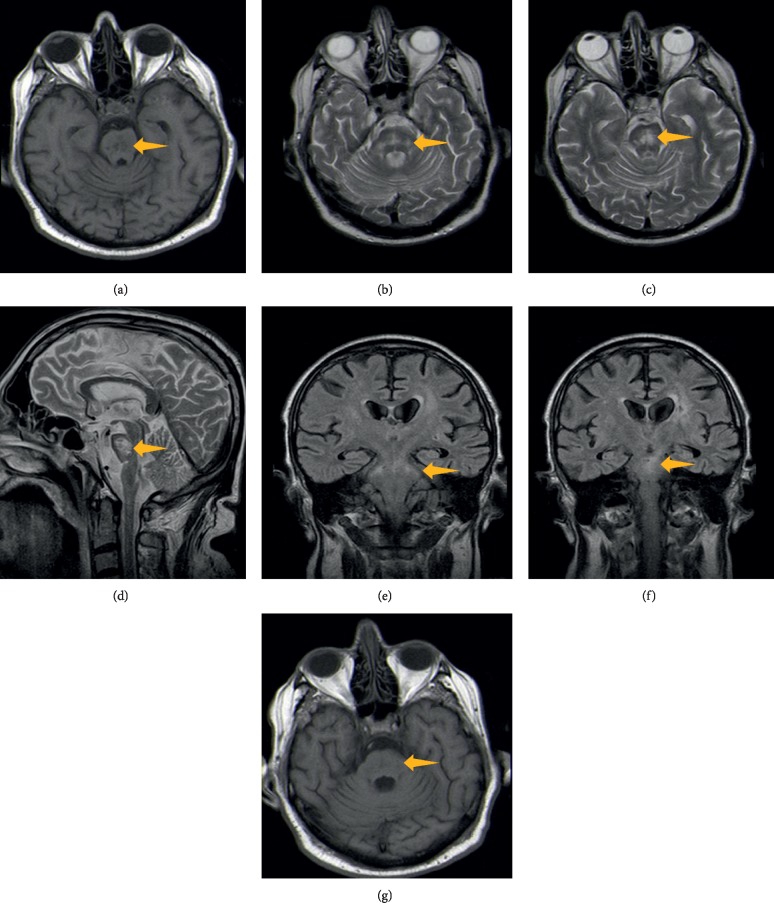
MRI images show central pontine T1 moderately hypointense areas (a), T2 and FLAIR areas of hyperintensity at the level of the pons (b, c, d, f), including an area trident-shaped of hyperintensity at the level of the pons on axial T2 (b) (typical appearances of the pons in osmotic demyelination syndrome), that extends to cerebellar peduncles (b, e); after gadolinium, there was no enhancement (g).
